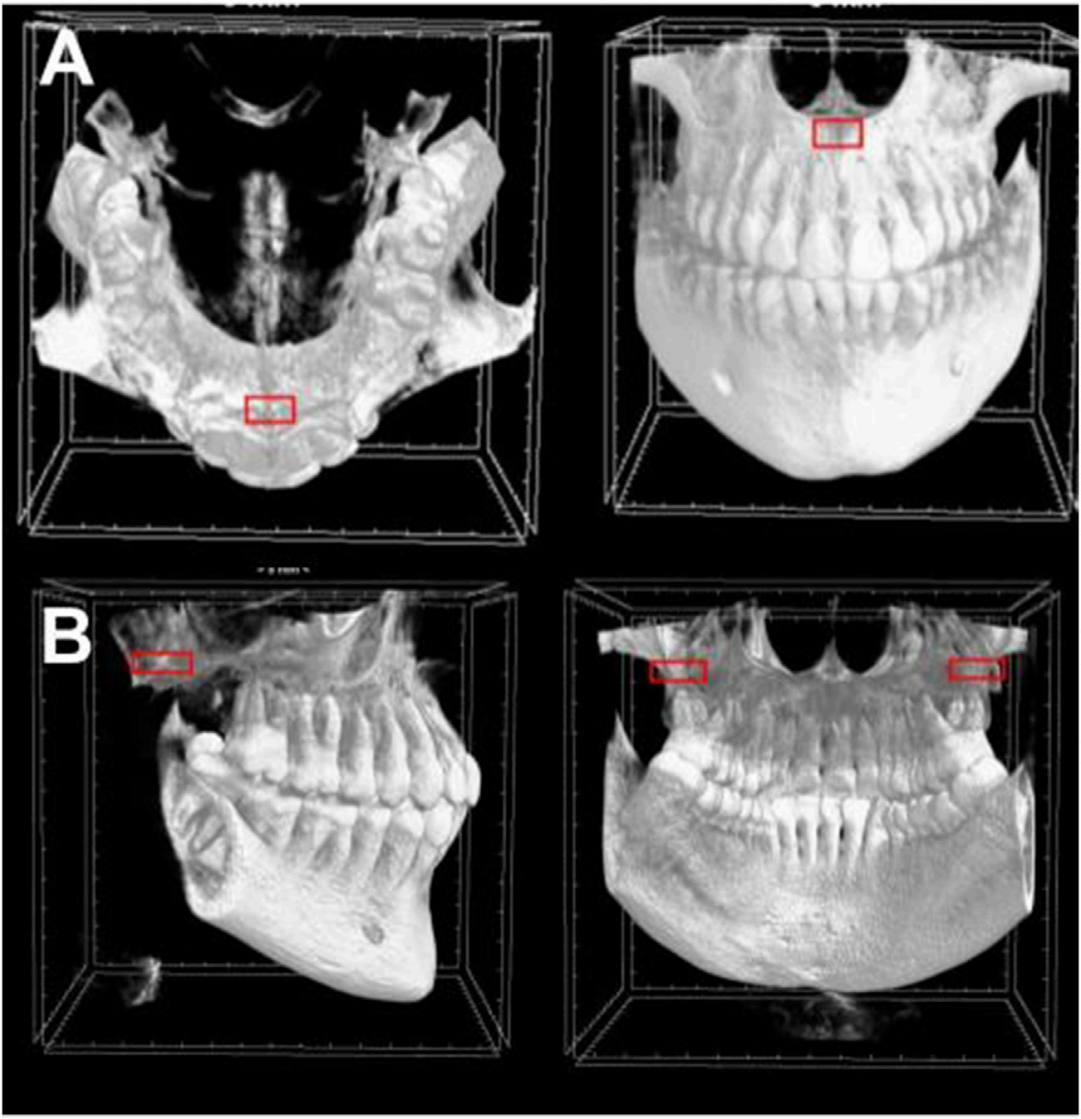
Regions of interest in CBCT sections. The region of interest box is demonstrated in red of (A) the incisive foramen and (B) the maxillary tuberosity.
